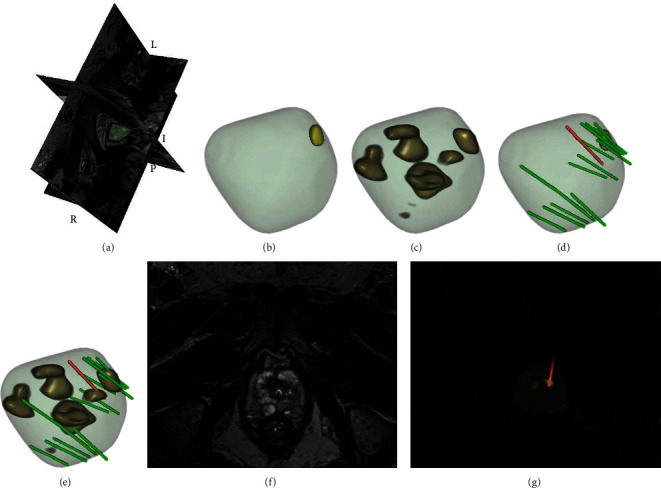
This figure presents an example of model detection: a 65-year-old man in the testing set with prostate-specific antigen of 5.5 ng/mL and PI-RADs of 4. (a) The patient's prostate is visualized in 3D coordinates for volume representation. (b) The patient's prostate surface and its ROI (yellow) outlined by radiologists. (c) The patient's prostate surface and its potential cancer distribution (dark yellow) generated by the WSUNet. (d) The patient's original ROI (yellow) and each biopsy core (Gleason grade ≥ 7 marked as red and <7 masked as green). (e) The ROI (dark yellow) detected by the model and each biopsy core (Gleason grade ≥ 7 marked as red and <7 masked as green). (f) A slice of the T2-weighted prostate MRI. (g) Slices with the detected ROI (brilliant yellow) and the positive biopsy core (red). In this meaningful example, targeted biopsy yielded no meaningful results, and the model presented a cancer distribution, part of which was validated by systematic biopsy.
